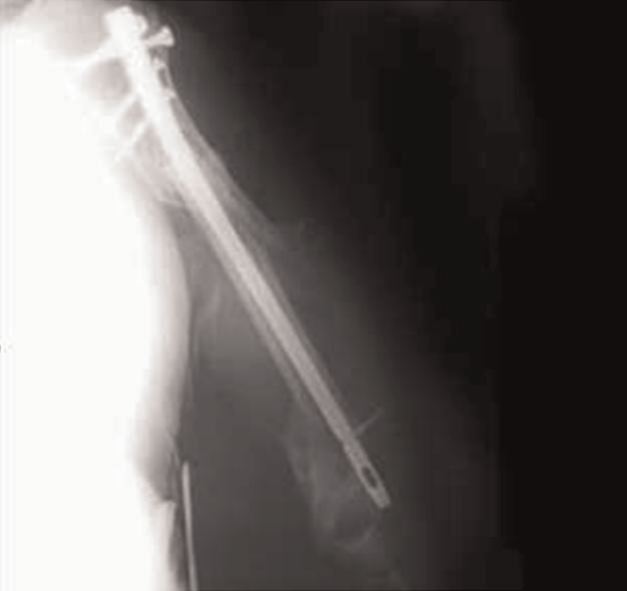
AP radiograph of the periprosthetic fracture of left humerus.
